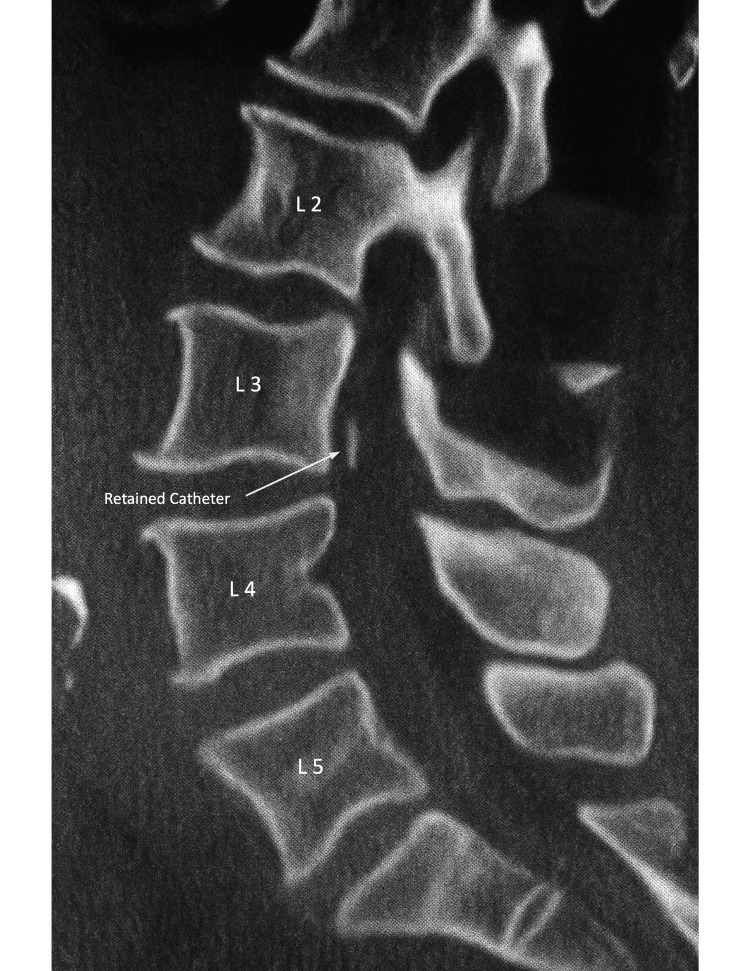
Lumbar spine CT Sagittal (lateral) view. Vertebral levels are labeled for orientation. The arrow indicates the retained catheter fragment within the intrathecal space.
